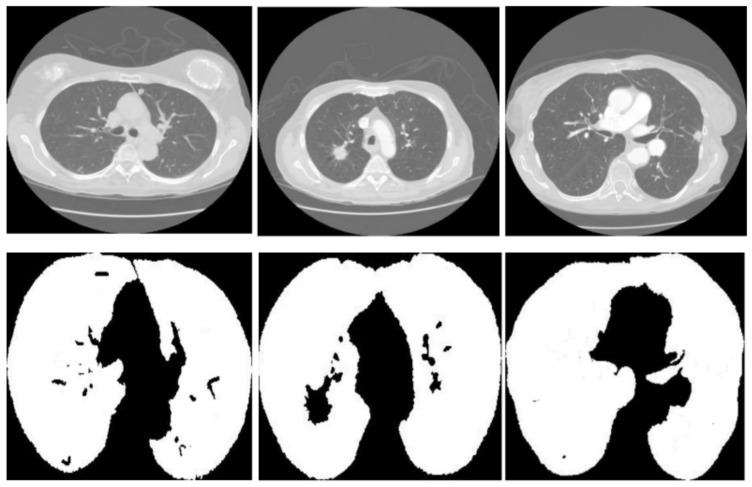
Comparative visualization of original CT scans and segmented lung regions by the proposed model.
